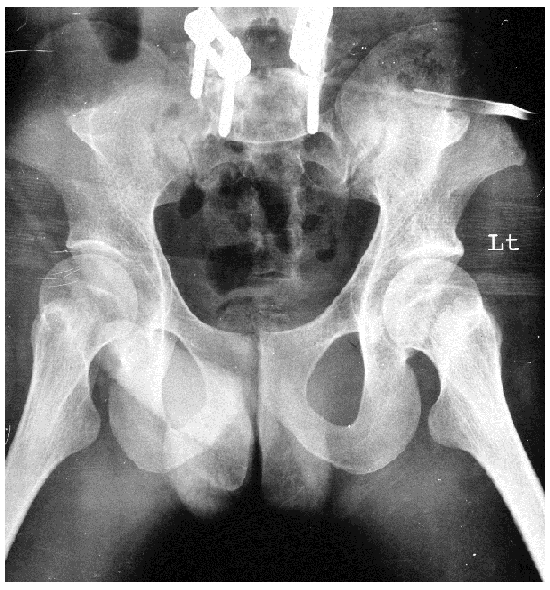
X-Ray of pelvis showing bilateral iliac horns
